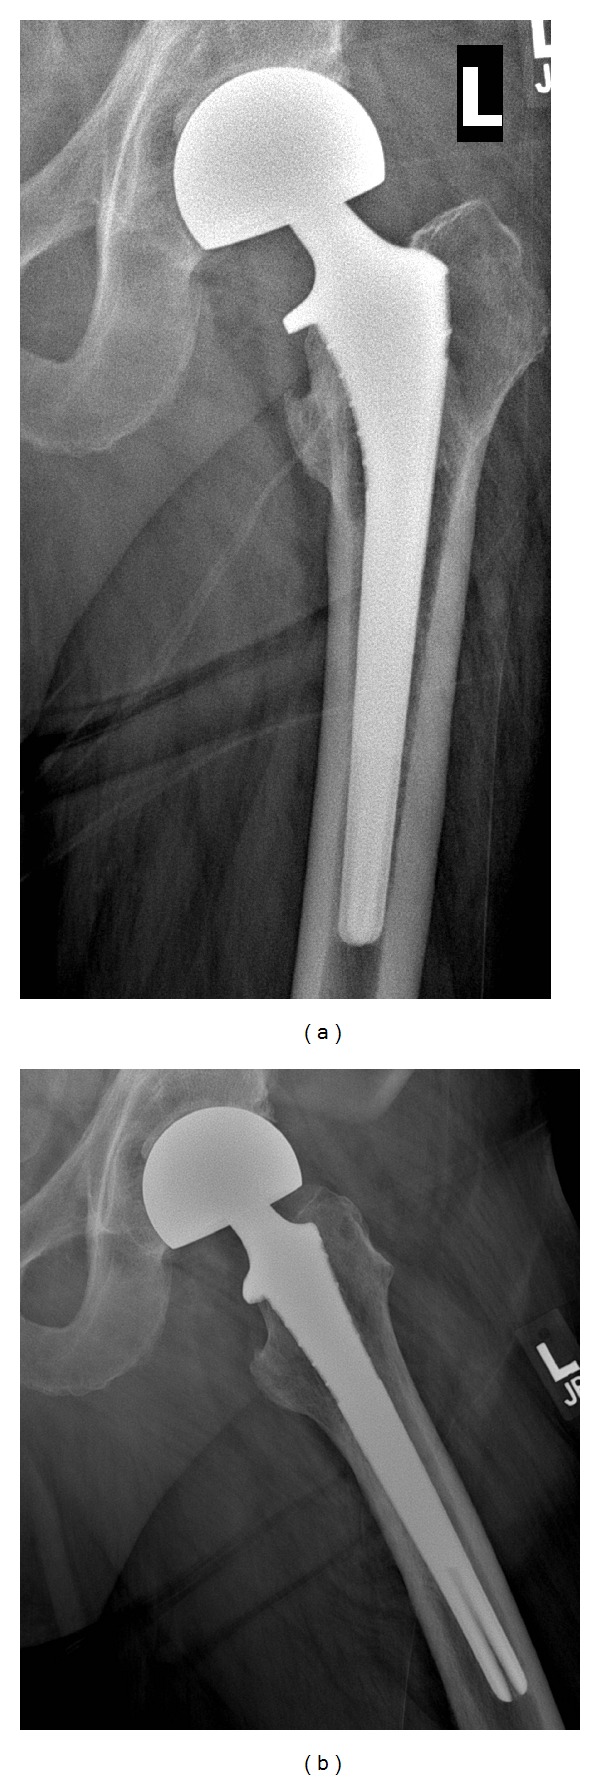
50-year-old construction worker—AP and lateral radiographs—10 years following hemiarthroplasty.
